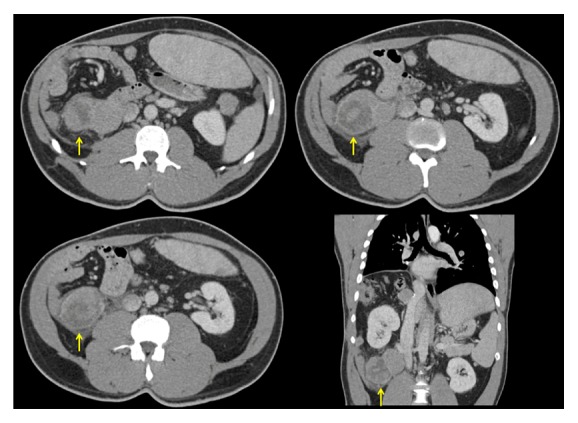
Abdomen and pelvis CT that shows a right-sided retroperitoneal recurrent mass prior to cryoablation.
